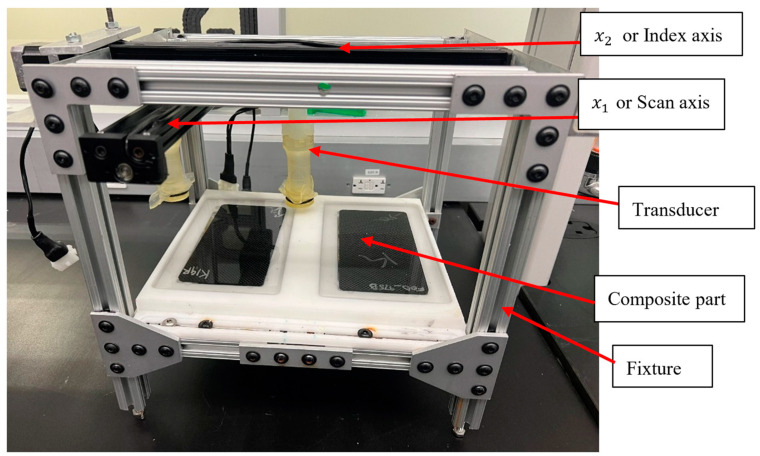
Portable housing of the ultrasonic system.
